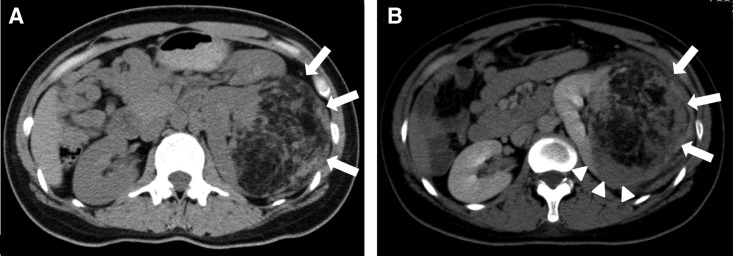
Computed tomography (CT) findings. (A) CT showed a ruptured left renal angiomyolipoma (AML; *arrows*) with a maximum diameter of 12 cm. (B) Enhanced CT showed a reruptured AML (*arrows*) with intratumoral hemorrhage (*arrowheads*).
